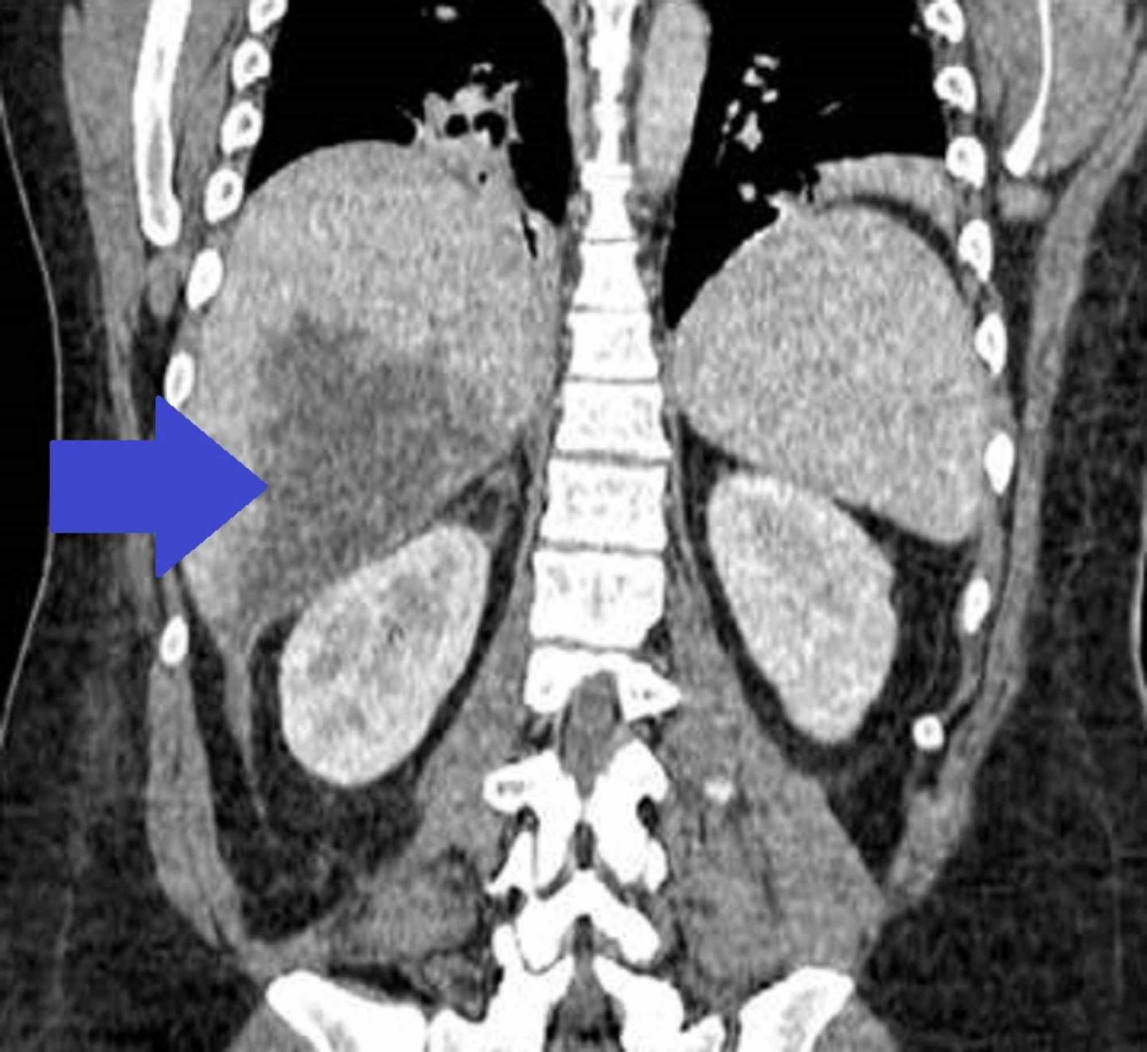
Computed tomography (CT) scan of the abdomen demonstrating a hypodense lesion in the right hepatic lobe
